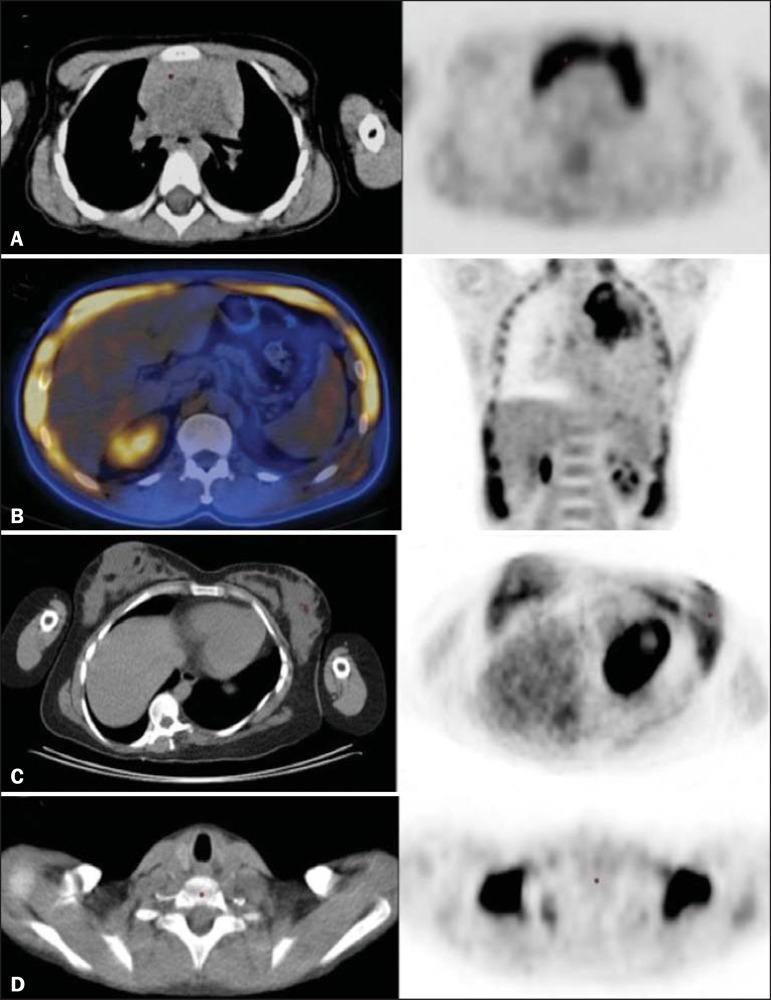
Causes of physiological FDG uptake in the thorax.*
